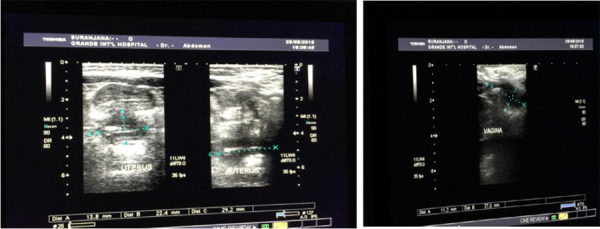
Post-operative USG. Postoperative ultrasonography (after 6 weeks) of the pelvic region revealed a normal size uterus with a small amount of fluid (27 × 4 mm) within the vagina.
